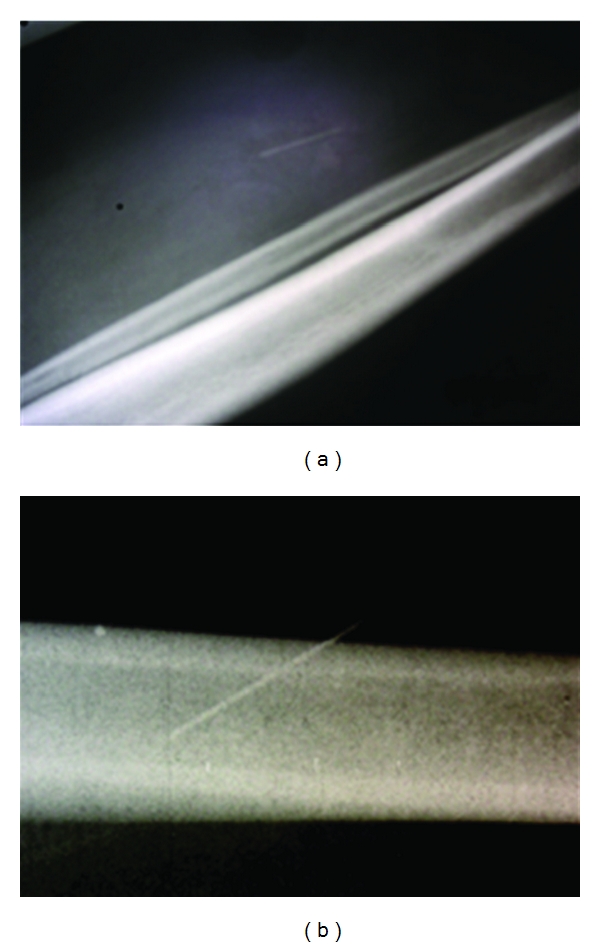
(a) and (b) Standard X-ray of the lower right leg showing presence of the sewing needle.
